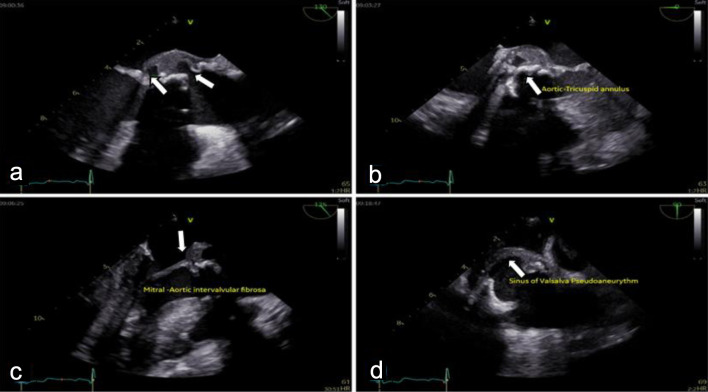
Transesophageal echocardiography (TEE) shows destructive prosthetic aortic valve endocarditis with peri-annular extension: (a) large mobile vegetation attached to the bioprosthetic aortic valve cusps, (b) extension of infection to the aortic annulus with paravalvular aortic root abscess formation, (c) destruction of the mitral–aortic intervalvular fibrosa (MAIVF) (arrow), and (d) a sinus of Valsalva pseudoaneurysm (arrow).
